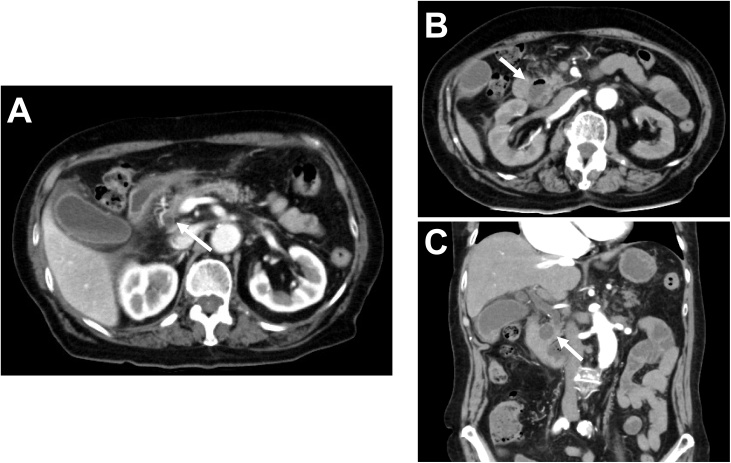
Contrast-enhanced axial and coronal CT of the abdomen and pelvis. Contrast-enhanced CT demonstrated (A) slight dilation of the CBD (arrowhead). (B) A coronal slice showing a large periampullary diverticulum containing fluid with wall thickening, mucosal enhancement, and surrounding inflammatory changes consistent with duodenal diverticulitis (arrowhead).
